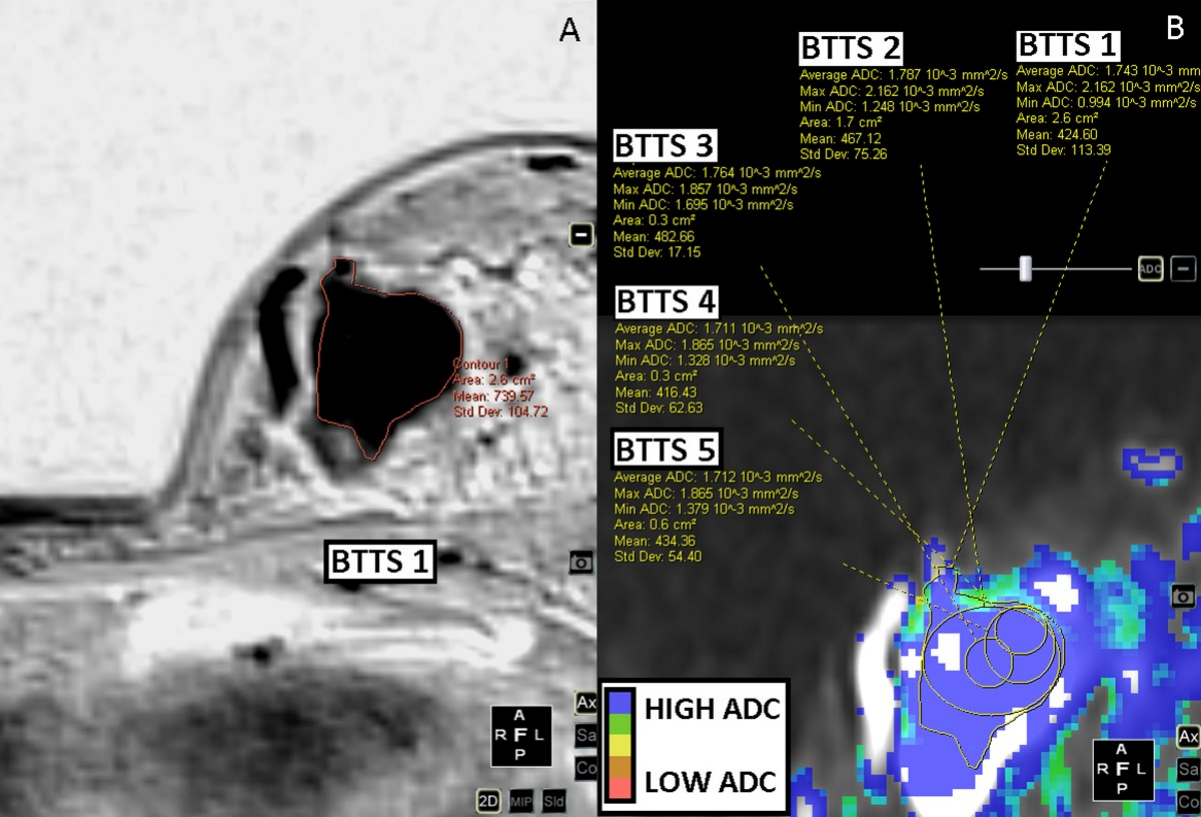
Benign phyllodes tumor of the left breast. DCE-T1 subtracted images (inverted) (A) and DWI b0-1000 images (B).
